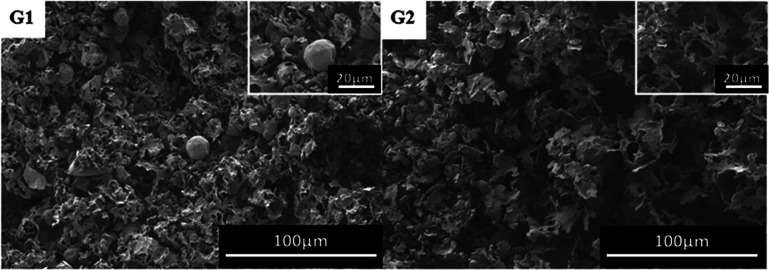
SEM images of poly(TFEMA–DVB) with different ratios of macro-CTA and initiator; (G1) macro-CTA/AIBN = 1, (G2) macro-CTA/AIBN = 2.
